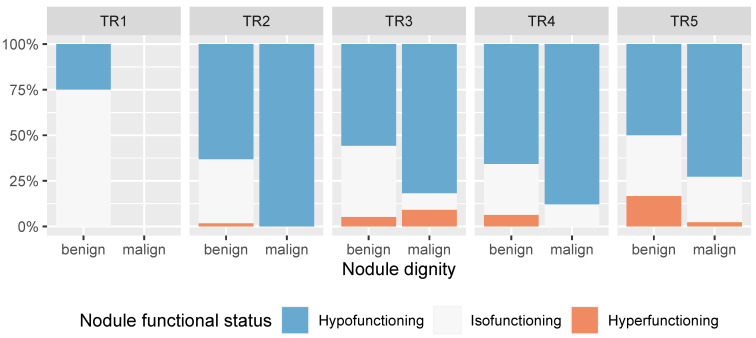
Barplot showing the relation of nodule dignity and functional status as assesed by scintigraphy, stratified by ACR-TIRADS category (*n* = 322 nodules in 208 patients). The proportion of hypofunctioning nodules is higher in malignant nodules in all ACR-TIRADS categories. Note that there are malignant hyperfunctional nodules.
